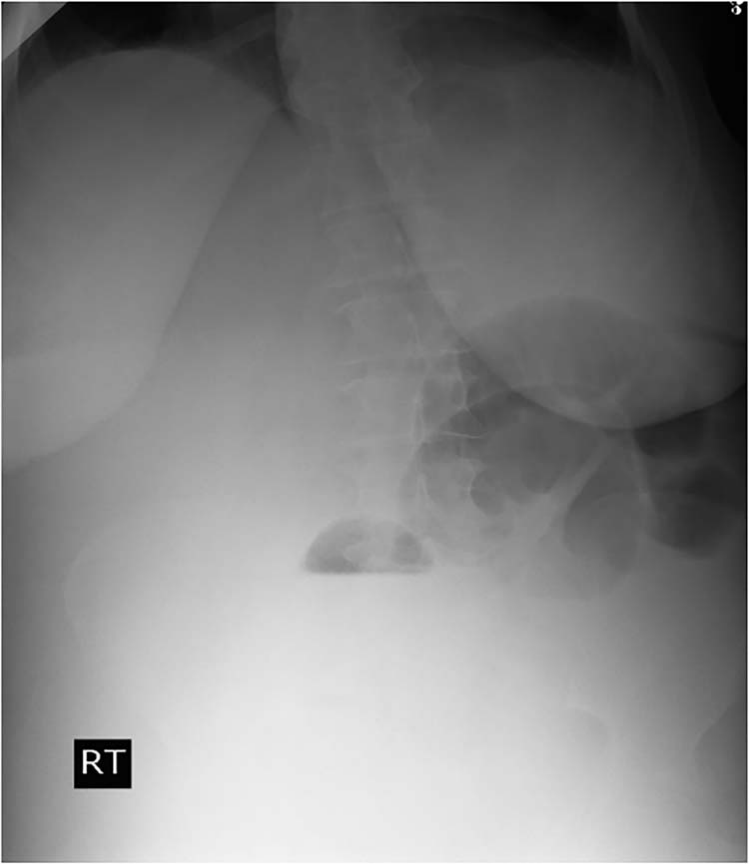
Plain abdominal X-ray demonstrated air-fluid levels of the small bowel.
